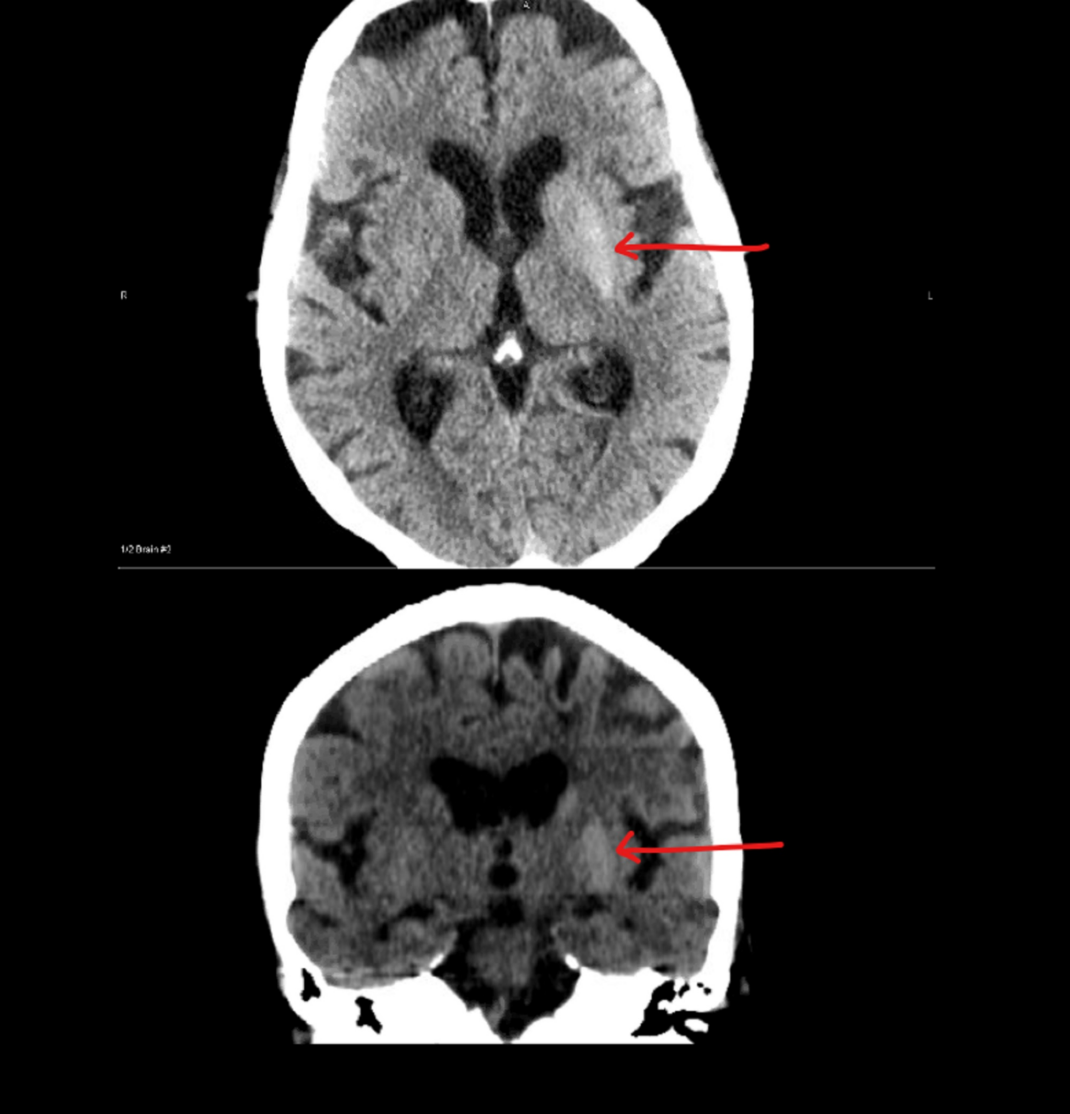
CT scan of head with out contrast showing asymmetric hyperdensity in the left basal ganglia.
